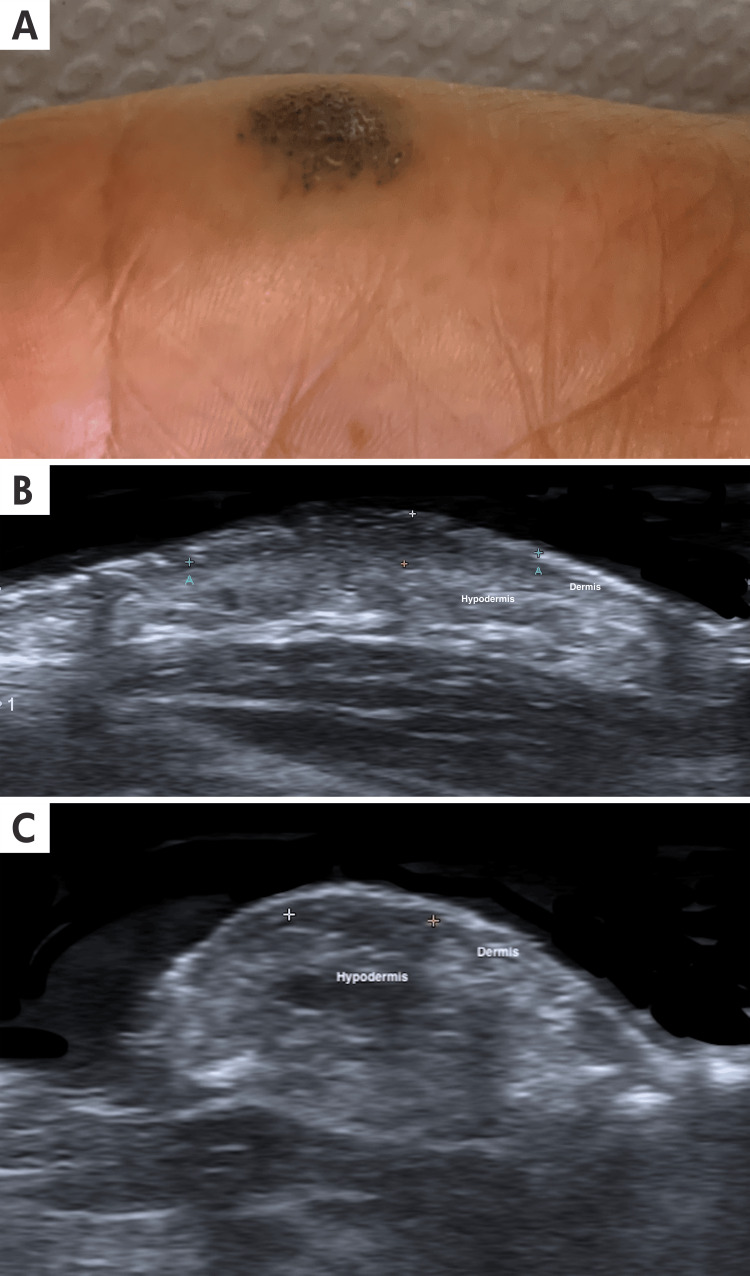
Dermatological ultrasound and clinical imaging of verrucous venous malformation A: A 51-year-old female patient with vascular dilations, papular in appearance, grouped to form a violaceous, rounded, irregular patch with a soft consistency and warty surface, approximately 1.5 cm in diameter, located in the left hypothenar region. B and C: The axial and longitudinal ultrasound view and grayscale ultrasound of the palmar aspect of the hypothenar left-hand show between calipers (+) a hypoechoic-like-band structure expanding the dermis and superficial hypodermis with poorly defined borders. There is some loss of definition in the dermo-hypodermis area.
